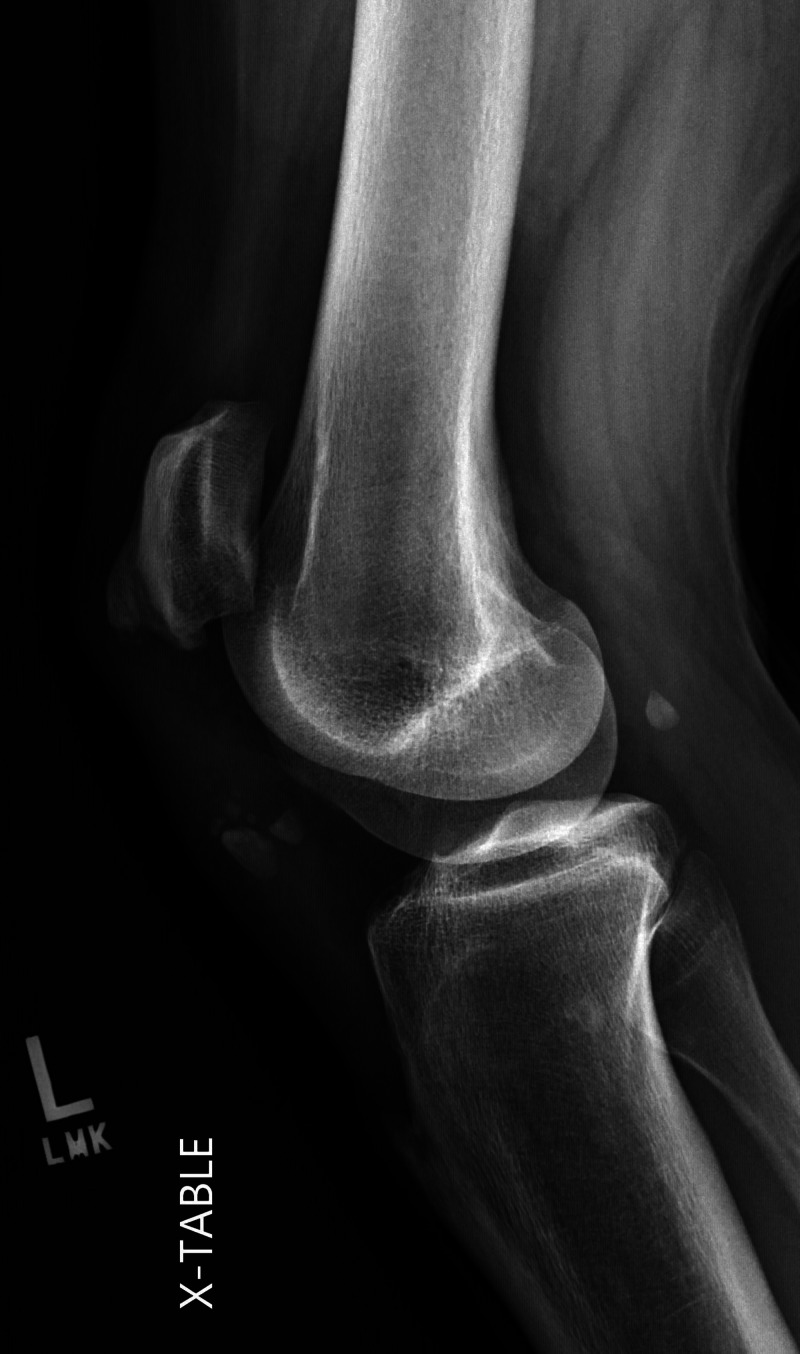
Lateral view radiograph of the left knee
